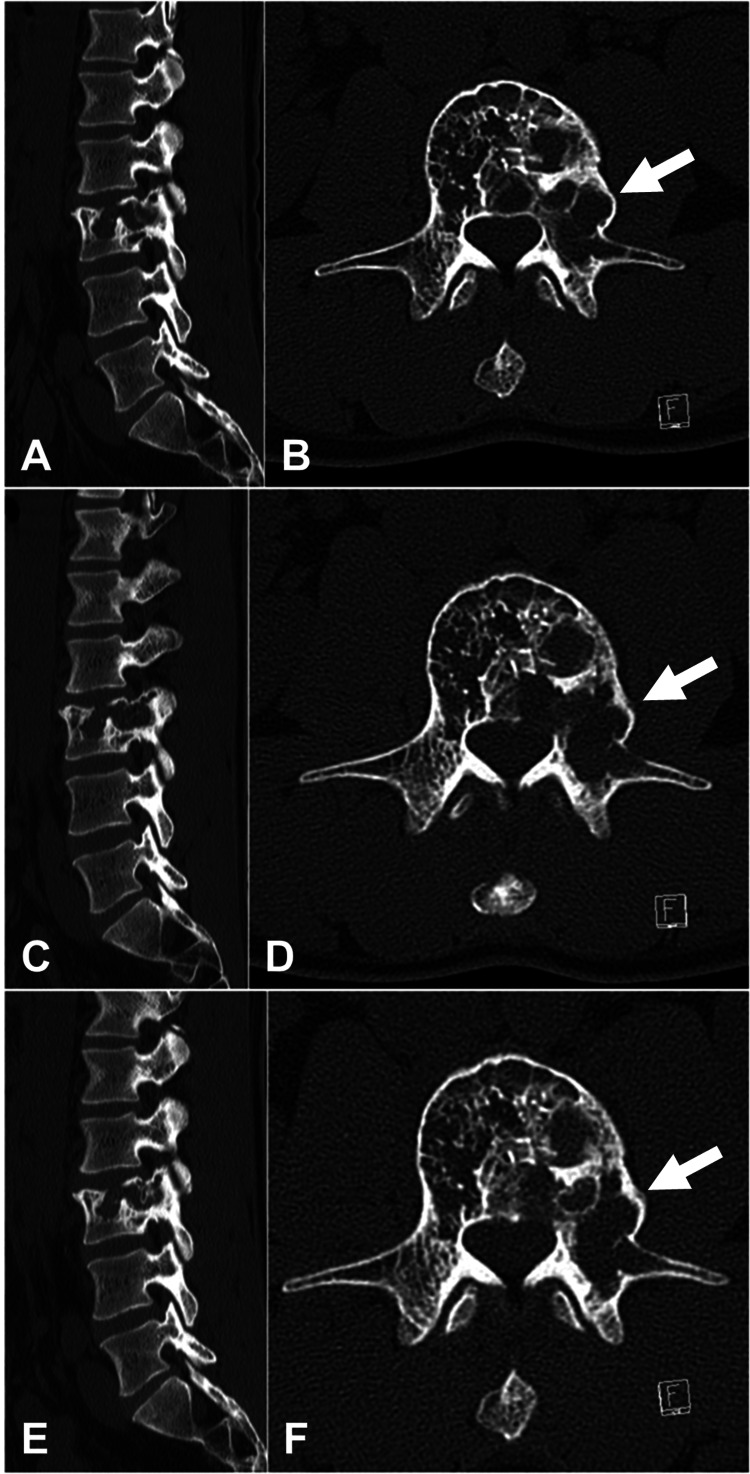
Reconstructed computed tomography. (A) At 16 weeks, decrease in the size and number of cysts, and most ossification of the lesion. (B) At 17 months, expansion of the erosive lesion. (C) After the re-treatment of denosumab had to be restarted, no evidence of recurrence on computed tomography. (A, C, and E) Sagittal view. (B, D, and F) Axial view.
